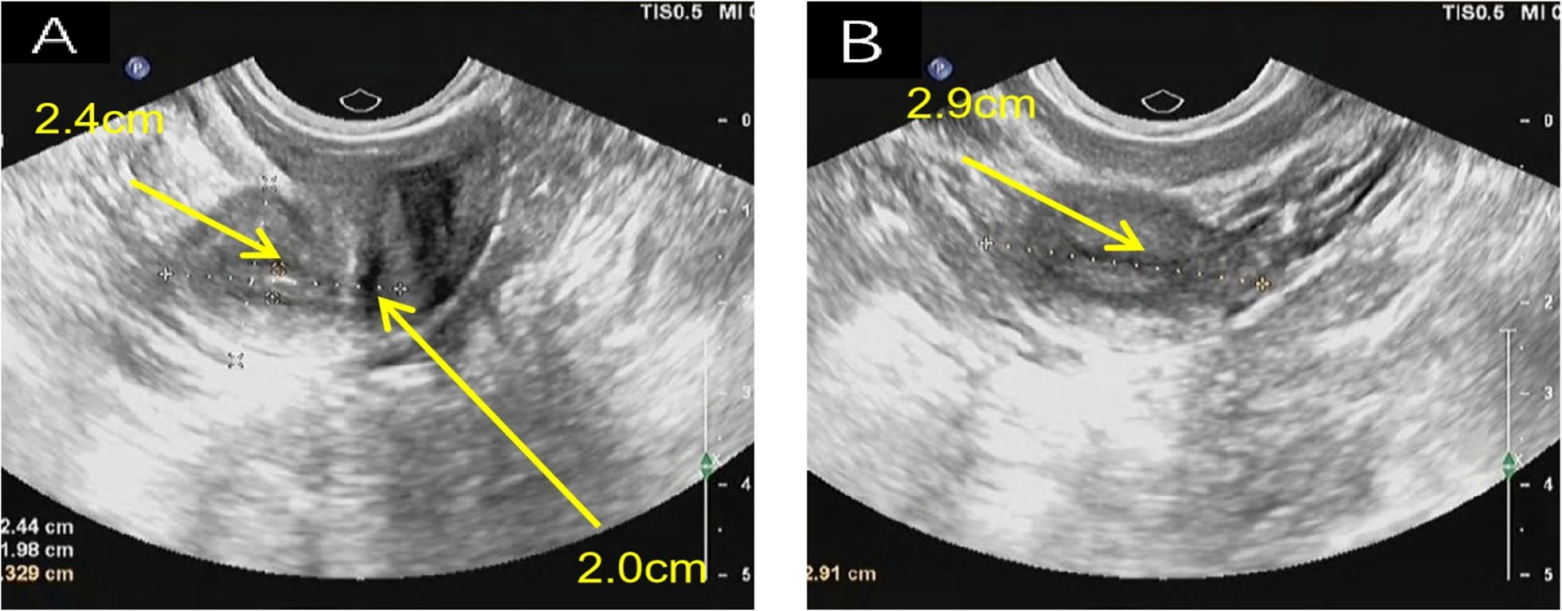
Gynaecological ultrasound images. A Longitudinal section of the uterus; B transverse section of the uterine body. Gynaecological ultrasound revealed an underdeveloped uterus. The length of the uterine body is approximately 2.4 cm, the diameter of the uterus is approximately 2.0 cm, and the diameter of the uterine body is approximately 2.9 cm. The cervix is relatively long, and the ratio of the length of the uterine body to the cervix is approximately 1:1. The endometrium is approximately 0.33 cm thick. Neither ovary was explored
